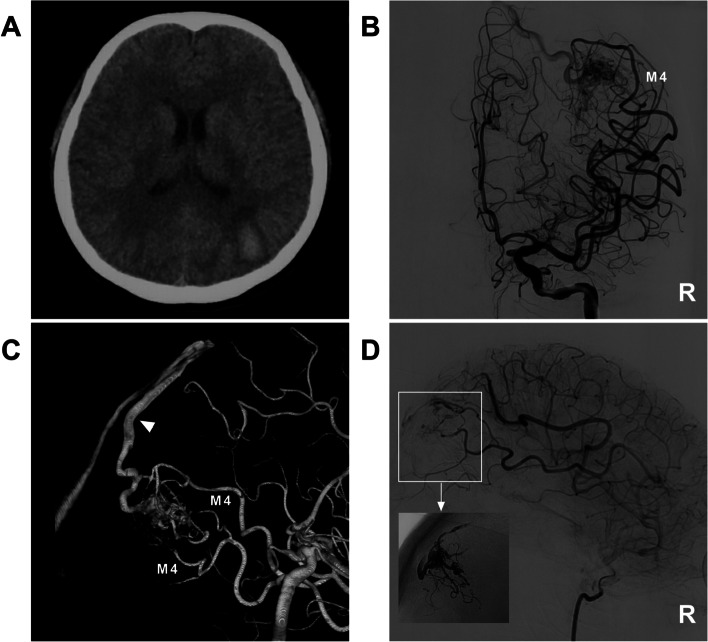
Curative EVT of a type IIIa BAVM. A CT showing hemorrhage in the left parietal lobe. B Anteroposterior angiogram of the right ICA showing that the BAVM was supplied only by the M4 segment. C Three-dimensional angiogram of the ICA showing that multiple branches of the M4 segment are involved as feeders, and only the superficial draining vein is observed (arrowhead). D Angiogram showing complete embolization of the BAVM (frame); the picture-in-picture shows the casting Onyx. Abbreviations: BAVM: brain arteriovenous malformation, CT: computed tomography, EVT: endovascular treatment, ICA: internal carotid artery, MCA: middle cerebral artery, M4: fourth segment of the MCA, R: right
